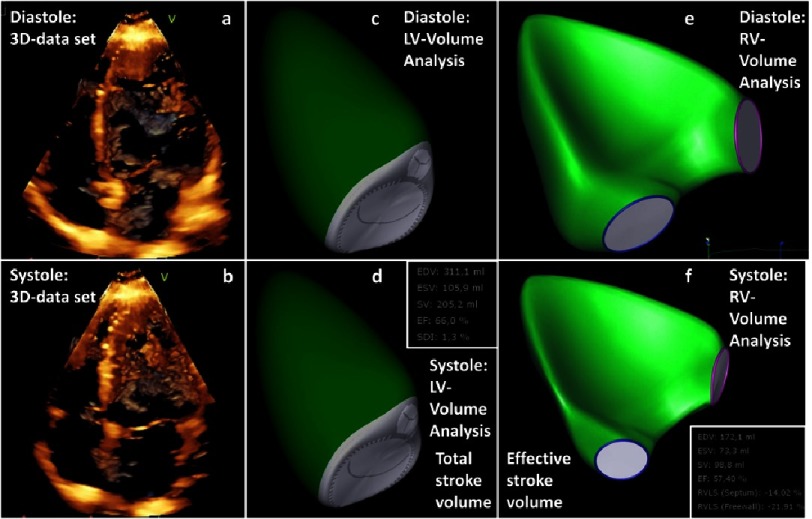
Determination of regurgitant fraction (RF) by 3D-echocardiography. 3D 4-chamber view during diastole (a) and systole (b); determination of LV volumes and total stroke volume (SV _t_) by post-processing of 3D data set during diastole (c) and systole (d); determination of RV volumes and effective stroke volume (SV_e_) by post-processing of 3D-data set during diastole (e) and systole (f); RF is calculated by RF[%] = [(SV _t_ − SV _e_)∕SV _t_] × 100.
